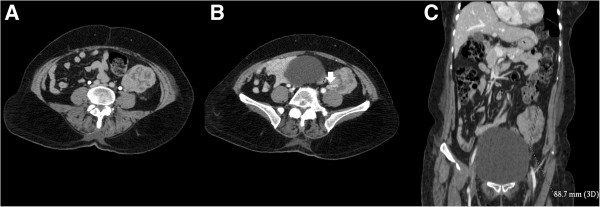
Pelvic CT scan-axial and coronal sections. CT scan of pelvis in arterial (A) and portal (B-C) phase, shows an inhomogeneous neoformation 65x51x88mm in correspondence of left ovary, with multiple necrotic areas within and a slow-enhancing after contrastum media administration. Figure 2B shows a vascular pedicle (white arrow) in the inferior portion of the lesion rising from left ovarian artery.
